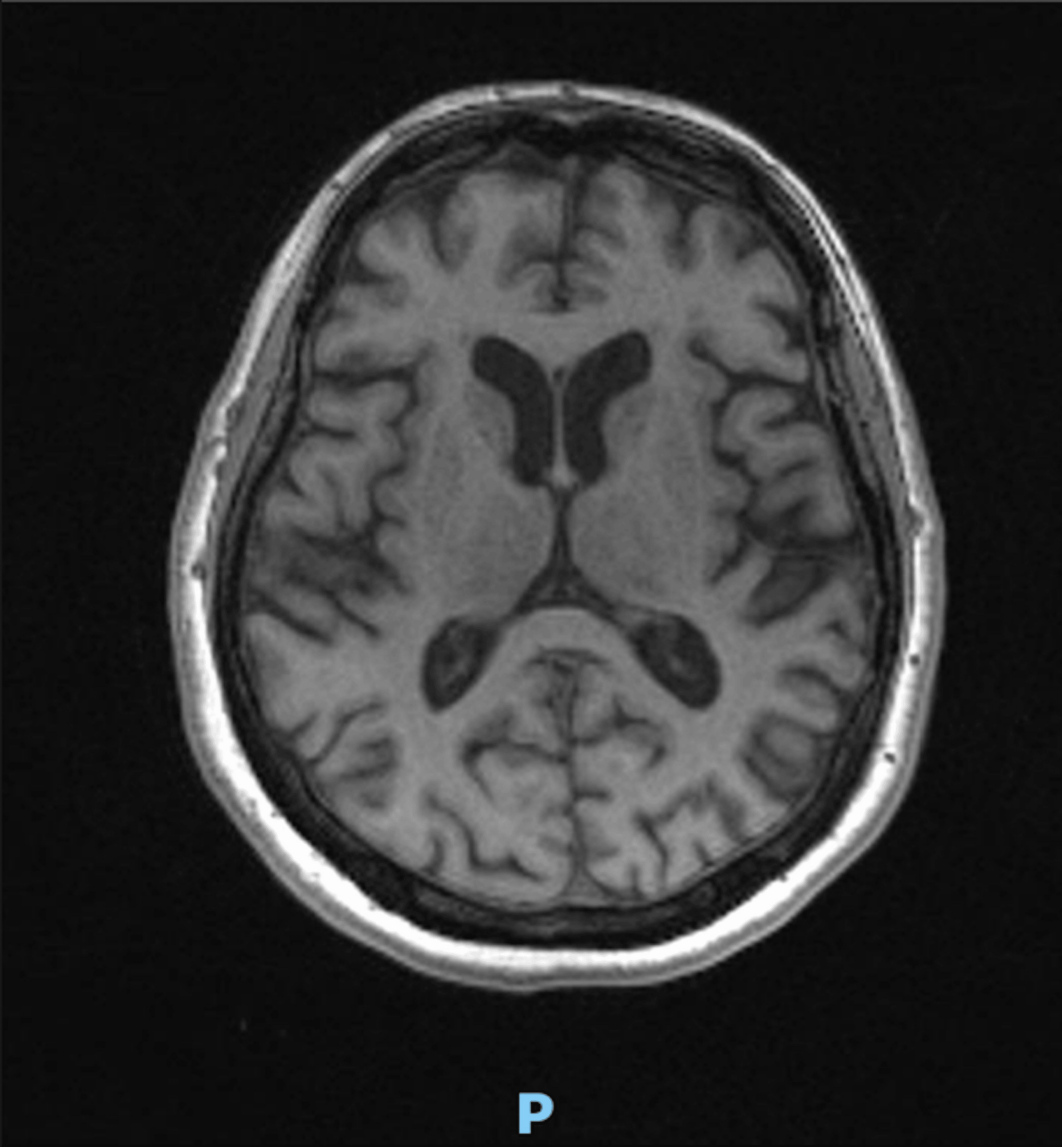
June 2020: MRI head showing possible mild chronic small vessel disease but no significant reduction in brain volume
